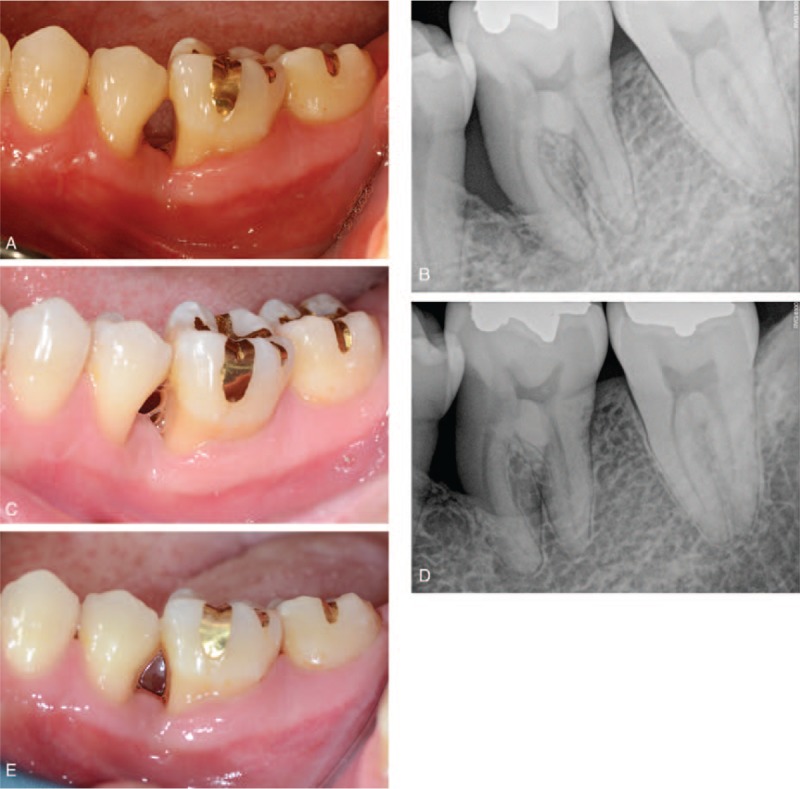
Follow-up check. (A) Seven-month postoperative buccal view showing healthy gingiva. (B) Seven-month postoperative radiograph showing increase in radiopacity. (C) One-year and 3-month postoperative clinical views indicating uneventful healing. (D) The radiograph at 1 year and 3 months postoperative, showing increased radiopacity around the left mandibular first molar. (E) Buccal view at 1 year and 9 months postoperative, showing healthy gingiva with regeneration of bony defect.
